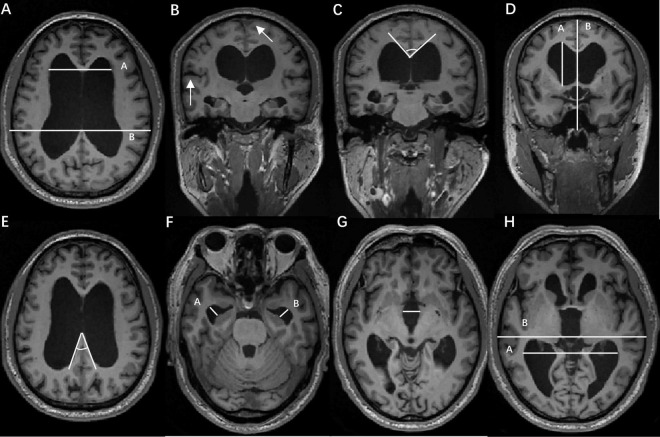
Radiological characteristics. (A). Evans Index (EI), the maximum width of the frontal horns of the lateral ventricles to the maximal internal diameter of the skull at the same level, as measured in axial magnetic resonance imaging (MRI) images; (B). Disproportionately Enlarged Subarachnoid Space Hydrocephalus (DESH), high convexity/midline tight sulci, and enlarged Sylvian fissures on MRI; (C). Callosal Angle (CA), the angle between the lateral ventricles on coronal MRI images within the anatomical structure of the corpus callosum; (D). z-Evans Index (z-EI), the maximum z-axial length of the frontal horns of the lateral ventricle to the maximum cranial z-axial length at the midline on the coronal plane, precisely located at the anterior commissure, z-EI = A/B. Brain per ventricle ratios (BVRs), BVRs = (B–A)/A; (E). Splenium of the corpus callosum angle (sCCA), the angle between the posterior part of the corpus callosum on axial MRI images; (F). Temporal horns, the width of the temporal horns of the left/right lateral ventricle in the anterior temporal lobe on axial MRI images; (G). Third ventricular width, the maximum width of the third ventricle on axial MRI images. (H). Lateral ventricle posterior angle ratio, the ratio of the maximum width of the posterior horns of the lateral ventricles, and the maximal internal diameter of the skull at the same level employed in axial MRI images.
